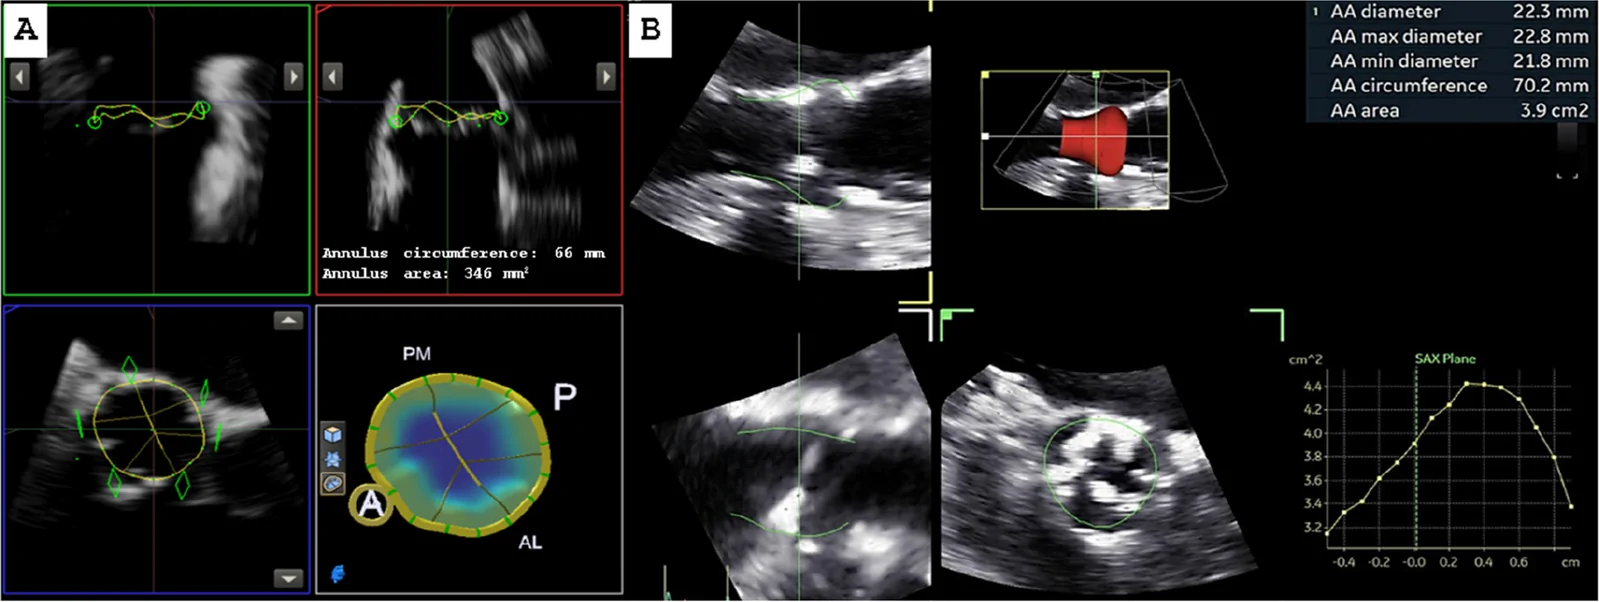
Indirect planimetry and semi-automated measurements of the aortic annulus on transesophageal echocardiography. A An example of indirect planimetry using mitral valve quantification software that uses the orthogonal long-axis view to identify the annular plane in its corresponding short-axis view, followed by provision of its planimetered area and circumference without hand-tracing. B New semiautomated aortic valve quantification software is available on cart that can automatically provide the annular dimension in terms of its diameter (maximal, minimal and averaged), area and circumference, after verification of the annular plane on 3D multiplanar reconstruction
